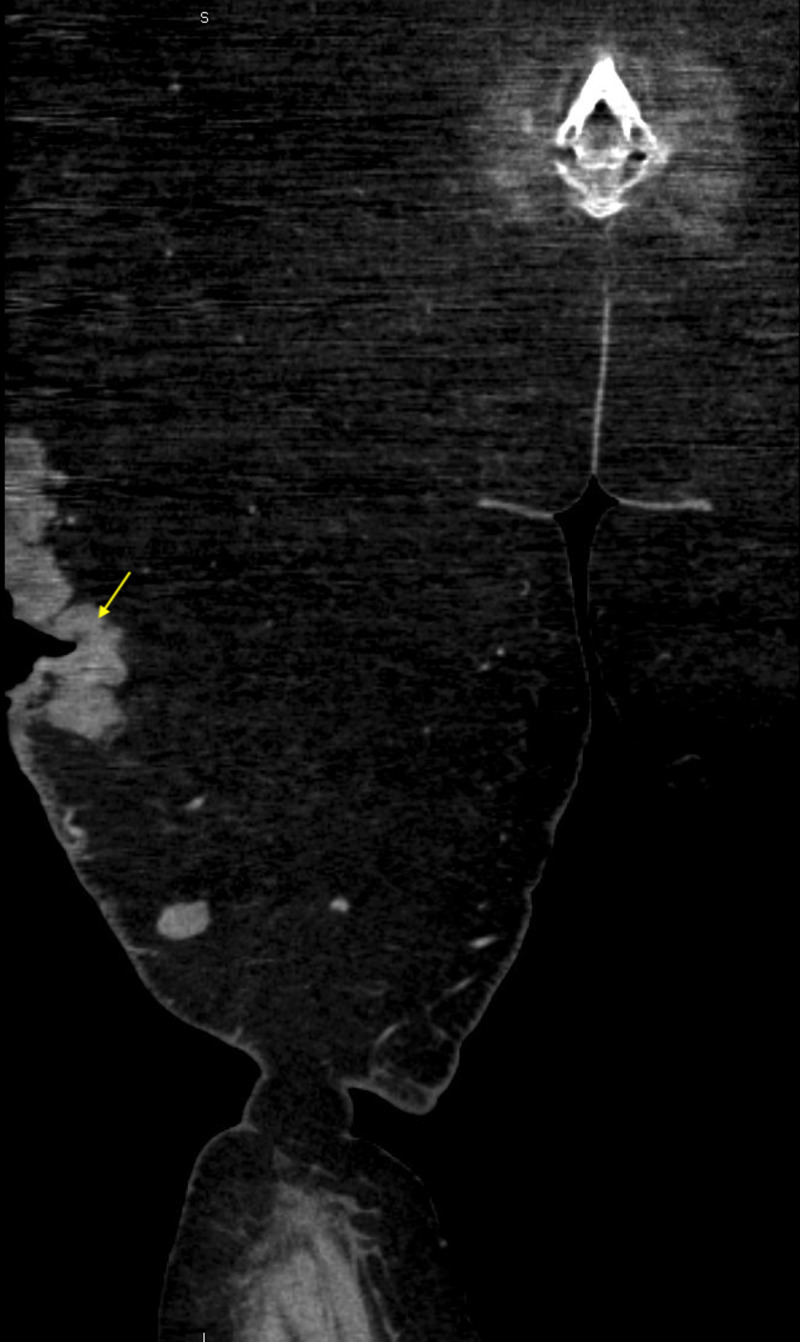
CT scan of the right lower extremity with contrast. Coronal section of the right lower extremity demonstrating subcutaneous angiosarcoma with associated tumor ulceration. Yellow arrow: Ulcerating subcutaneous angiosarcoma
